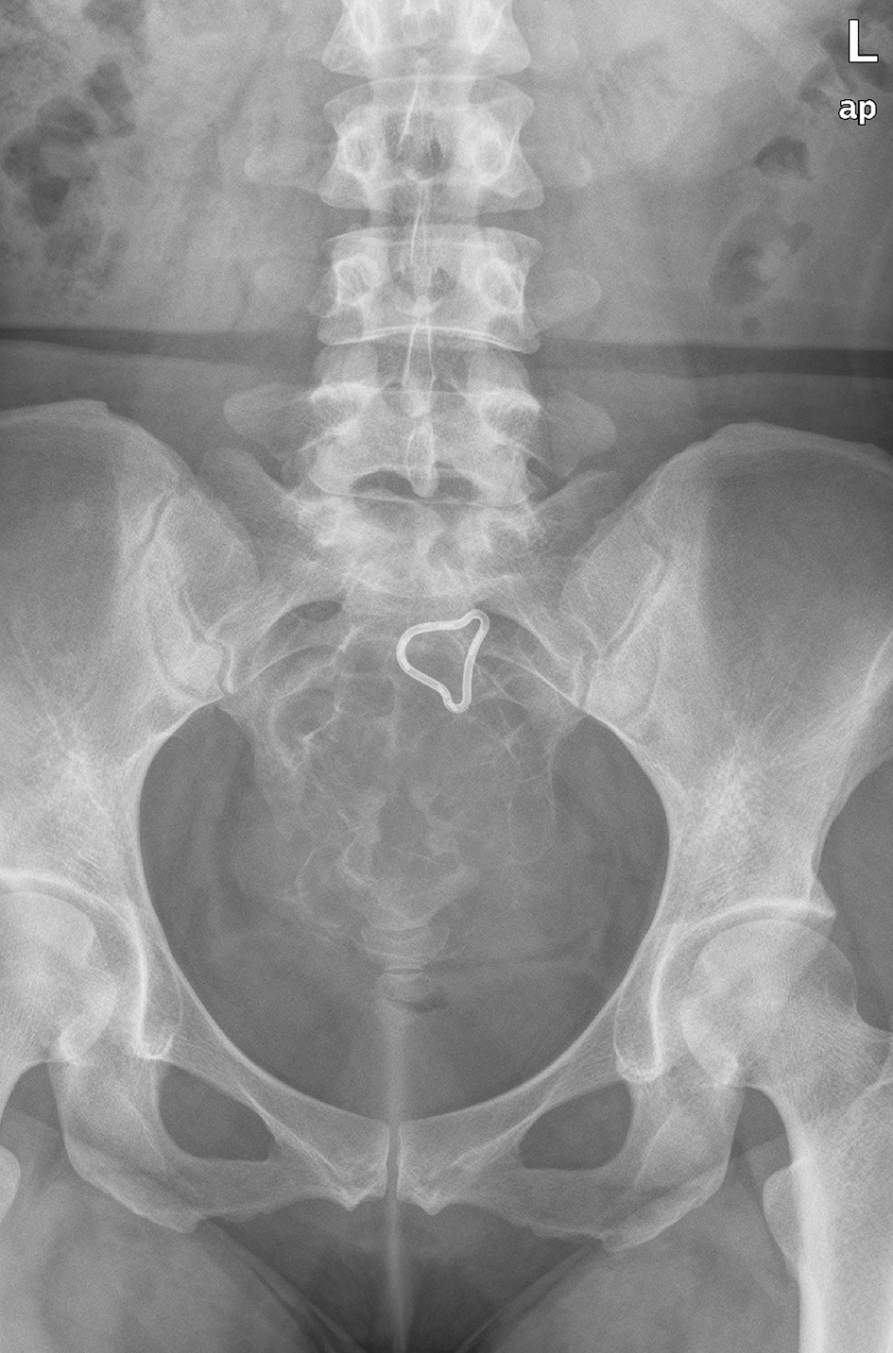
Radiograph shows markedly expansile, well-marginated, mixed lytic and sclerotic lesion of the sacrum.
